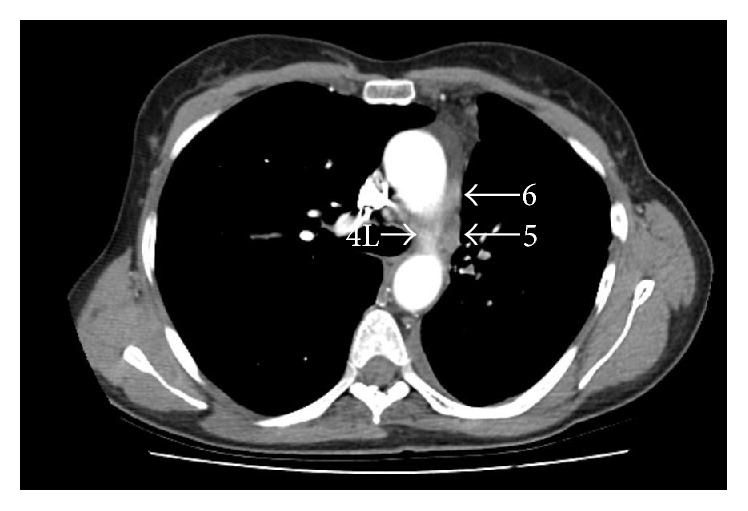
Computed tomography chest scan at the level of IASLC stations 4L, 5, and 6 (white arrows).
